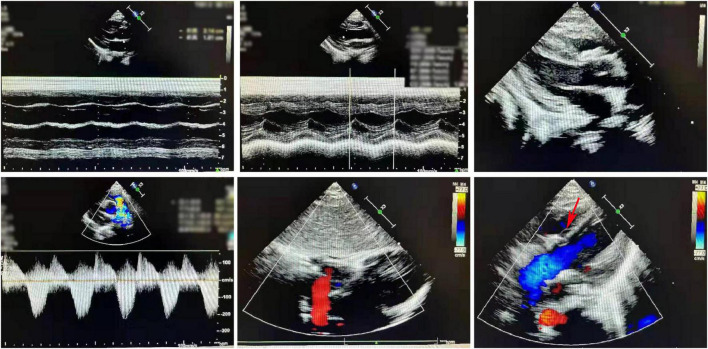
A representative image of the cardiac ultrasound (postoperative) shows the normal internal diameter of each chamber, low septal motion amplitude, and strong plaque echoes around the septum. The right ventricular outflow tract is not obstructed. The muscle bundles are not hypertrophic. The internal diameter of the pulmonary artery was normal.
